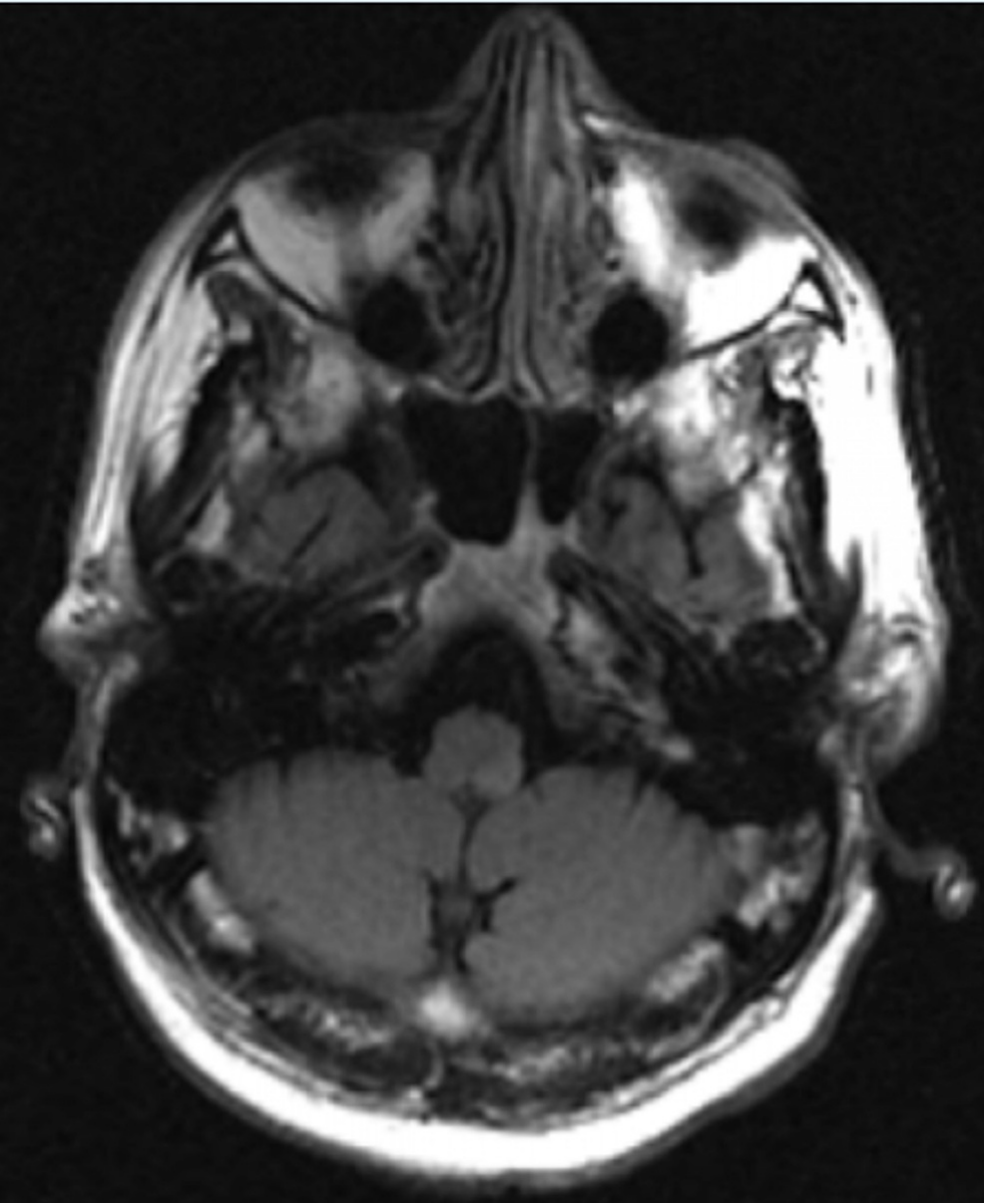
Initial MRI of the brain showing no significant hyperintensity on T2-FLAIR T2-FLAIR: T2-weighted fluid-attenuated inversion recovery
